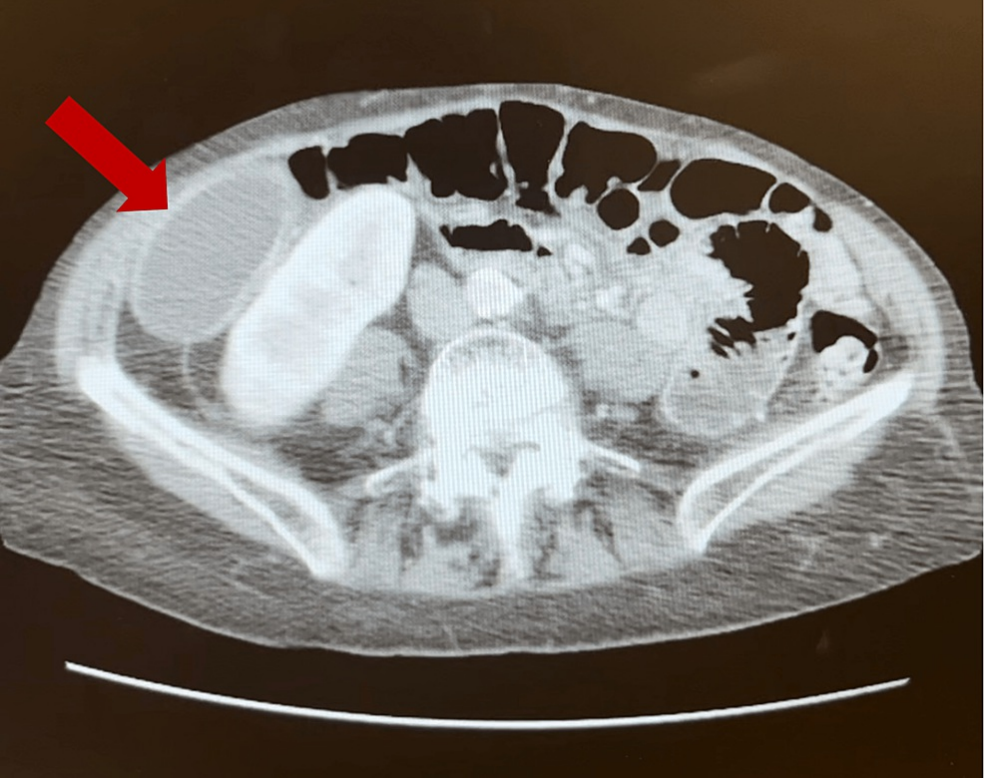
CT showing gallbladder in the right paracolic gutter/lateral to the right kidney
